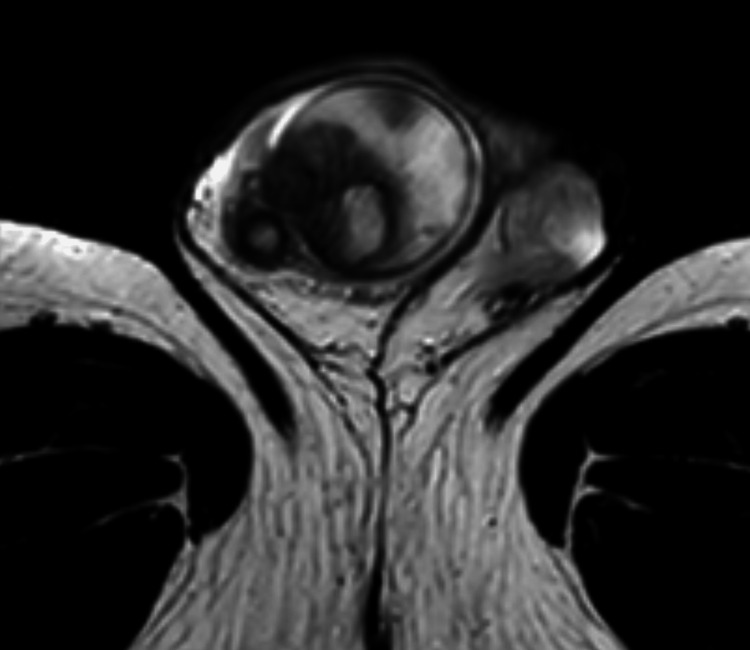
T2-weighted MRI of the pelvis at hospitalization Transversal view showing right testicular lesions with central hyperintensity and peripheral hypointensity. The right epididymis shows tubular dilatation with central hyperintensity and peripheral hypointensity
